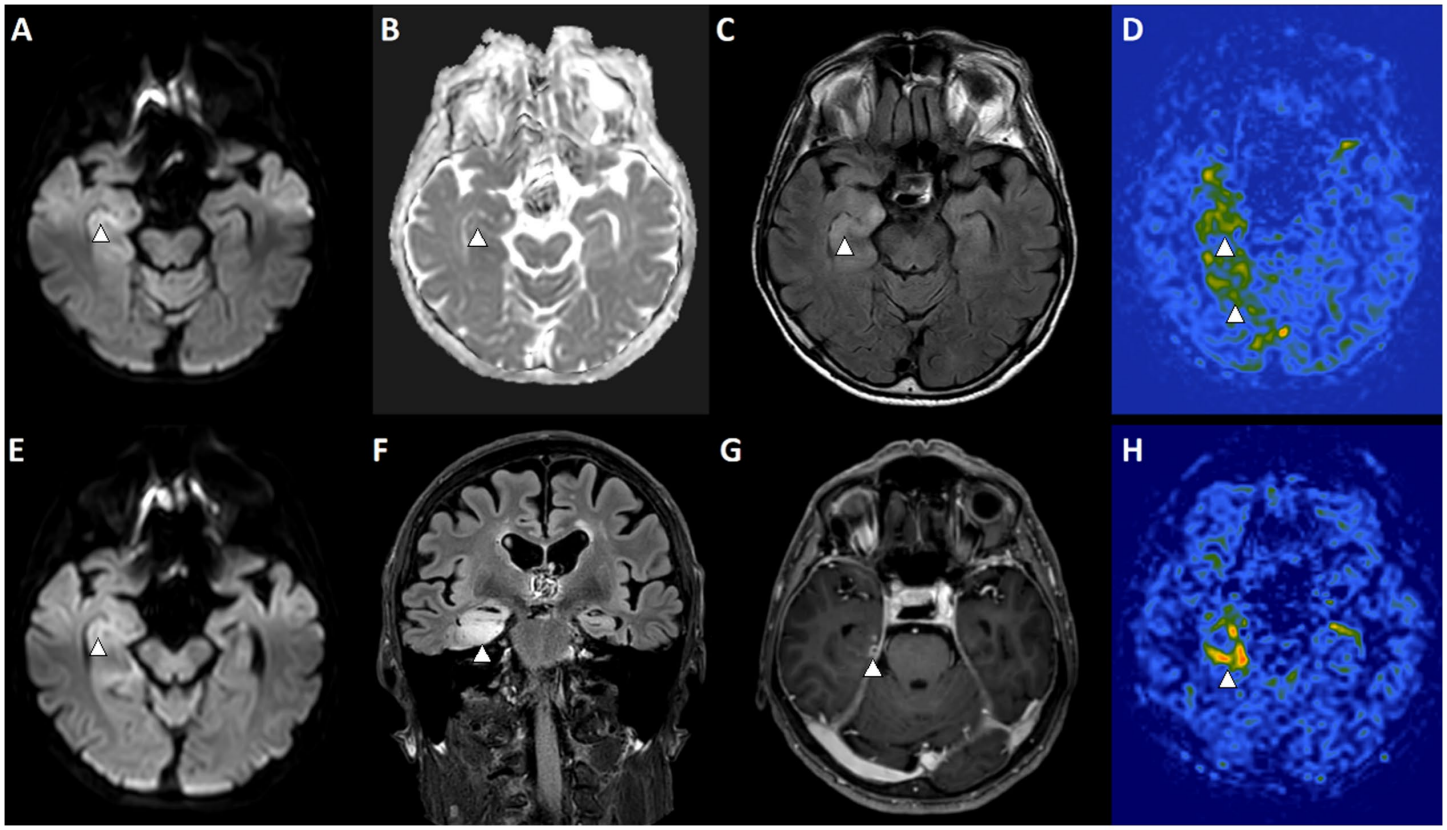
Overlap of PMA-like imaging features and encephalitis-related MRI findings in a patient with status epilepticus. Baseline MRI (A–D) and 7-day follow-up MRI (E–H) are shown. (A) Axial diffusion-weighted imaging (DWI) demonstrates a subtle diffusion abnormality in the right hippocampus/mesial temporal region (arrowhead). (B) The corresponding apparent diffusion coefficient (ADC) map shows only minimal signal reduction, consistent with a mild diffusion restriction pattern (arrowhead). (C) Axial FLAIR reveals right mesial temporal hyperintensity involving the hippocampus and uncus, compatible with edema/inflammation (arrowhead). (D) Arterial spin labeling (ASL) shows concordant hyperperfusion in the right hippocampal/parahippocampal region, consistent with PMA-like perfusion changes (arrowheads). (E) Follow-up DWI after 7 days demonstrates persistent subtle diffusion abnormality in the right hippocampus (arrowhead). (F) Coronal FLAIR shows marked progression of right mesial temporal edema (arrowhead). (G) Post-contrast T1-weighted imaging demonstrates new enhancement in the right mesial temporal region, supporting an encephalitis pattern (arrowhead). (H) Follow-up ASL demonstrates spatially congruent hyperperfusion relative to the FLAIR abnormality, consistent with persistent PMA-like perfusion features (arrowhead).
